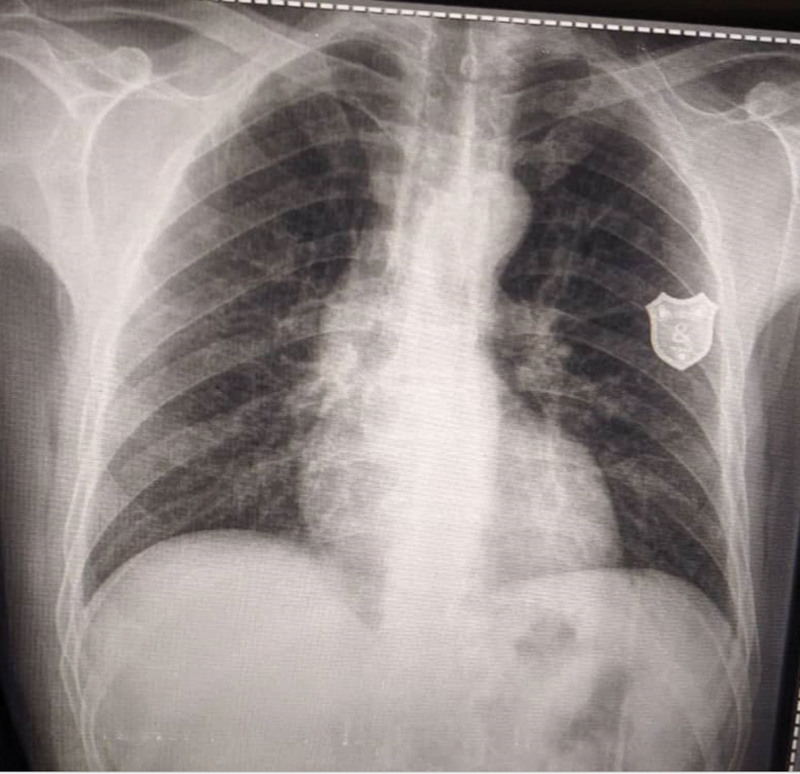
Chest X-rays show normal findings with no evidence of rheumatoid nodules.
